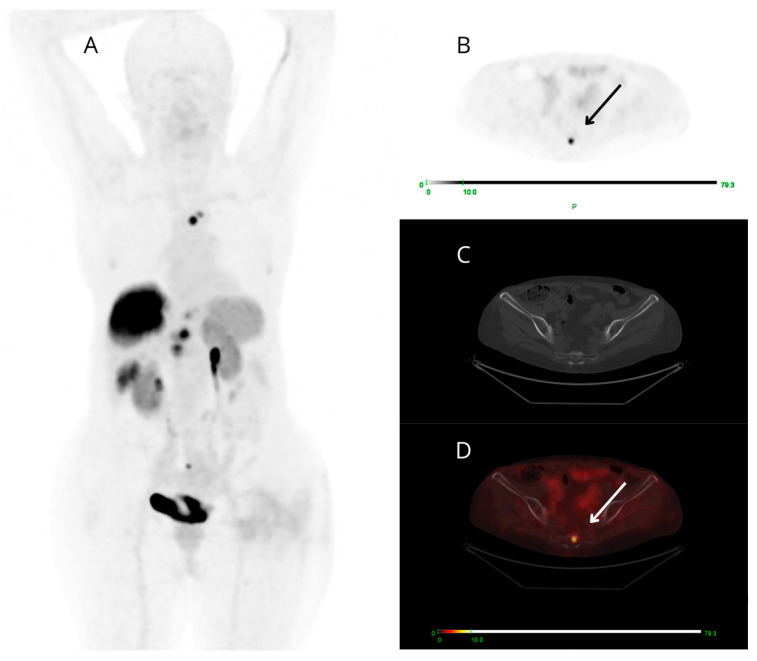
Maximum intensity projection (MIP) (A), PET (B), CT (C), and fused PET/CT (D) transaxial images of a patient studied for restaging of metastatic G3 NET, likely of pancreatic origin. [^68^Ga]Ga-DOTANOC PET shows high and focal uptake at the coccyx (arrows). The uptake area does not correspond to clear morphologic alterations on low-dose CT.
